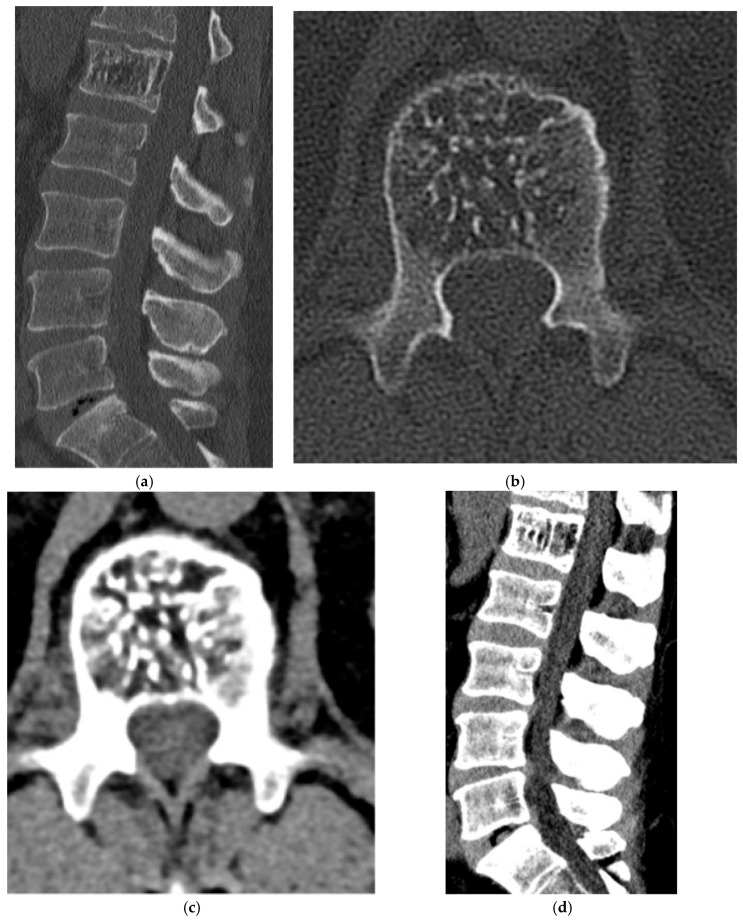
Hemangioma. (a) Sagittal CT bone window, hemangioma in the L1 vertebral body with the characteristic “corduroy” appearance. (b) Axial CT bone window, classic “polka dot” appearance of a hemangioma with the dots representing intact trabeculae. (c) Axial and (d) sagittal CT soft tissue window: intralesional fat is typical in hemangiomas.
